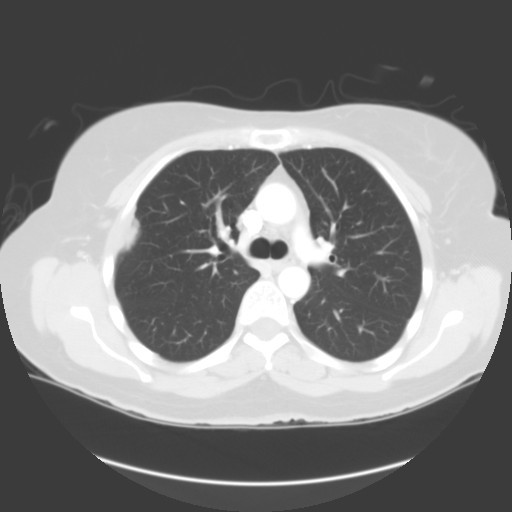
Chest CT obtained February 2006 showing pleural accumulation of mucinous adenocarcinoma.
